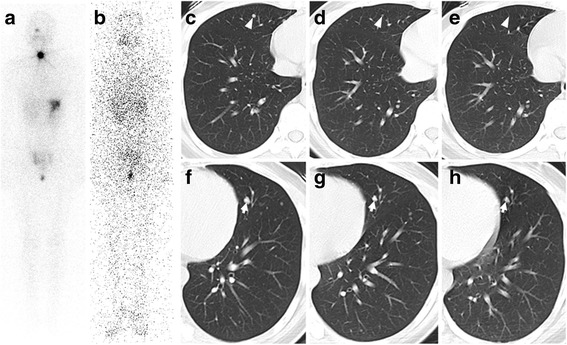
Imaging examinations. Whole body scintigraphy 3 days post the two doses of ^131^I (a 100 mCi for remnant ablation; b 200 mCi for cancer therapy) showed that there were no ^131^I–avid foci in the bilateral lungs (b). Diagnostic CT imaging (c and f, 3 months before getting pregnant, the maximum diameters of the largest lesions in the right and left lung were 3.75 mm and 6.66 mm, respectively; d and g, 21 months post cesarean section, the maximum diameters of the largest lesions in the right and left lung were 1.83 mm and 5.34 mm, respectively; e and h, 30 months post cesarean section, the largest lesion in the right lung was not seen and the maximum diameter of the largest lesion in the left lung was 4.88 mm) revealed shrinkage of metastatic foci without new lesions. Arrowhead, the largest lesion in the right lung; Arrow, the largest lesion in the left lung
